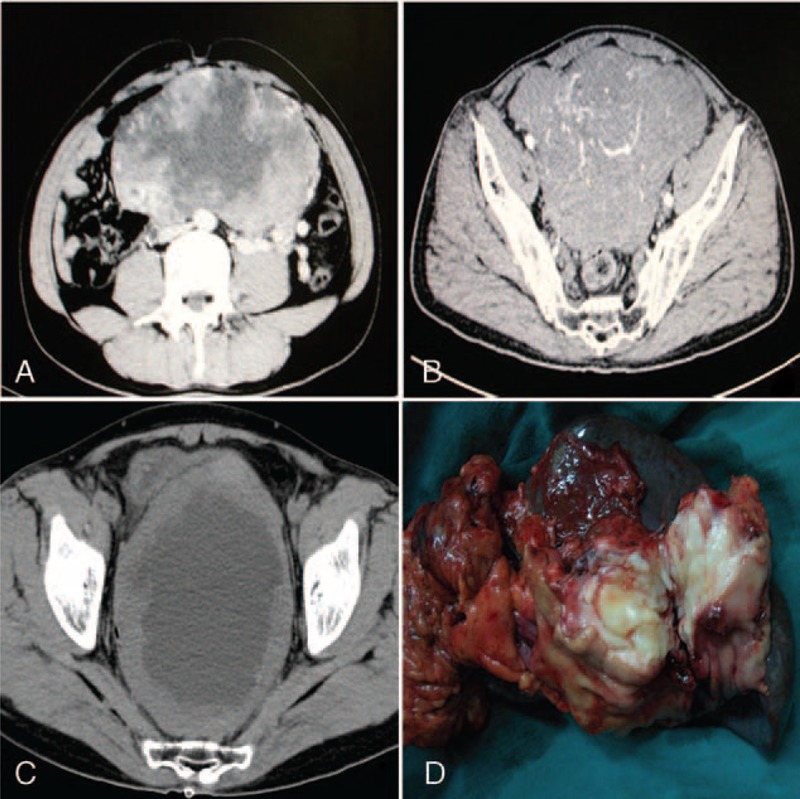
The computed tomography (CT) scan showed a giant tumor located in the abdominal cavity with a size of 22 cm × 16 cm (A). This patient underwent surgery after IM treatment, but eventually experienced tumor recurrence within 14 months postoperatively. Huge mass located in the pelvic cavity was observed by CT scans (B, C). The operative photo showed that the patient underwent proximal gastrectomy combined with splenectomy, which was attributed to the density adherent to the spleen (D).
